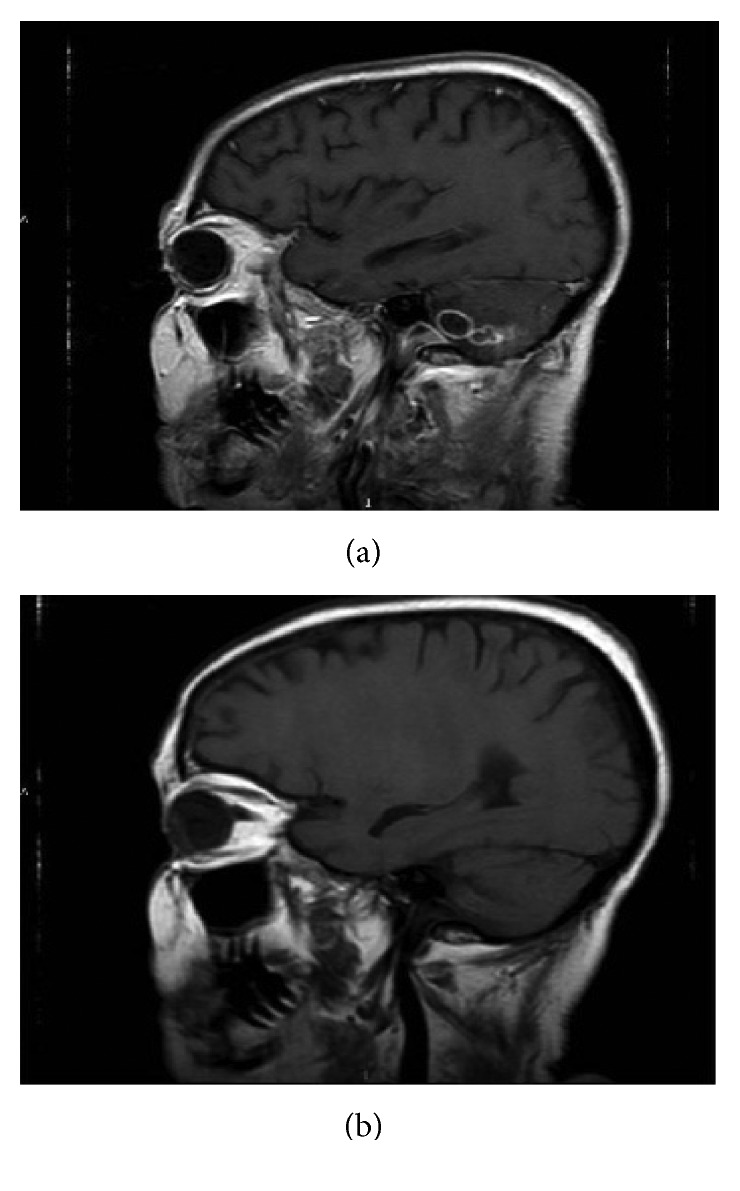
(a) MRI before starting albendazole. (b) MRI after 10 days of albendazole.
